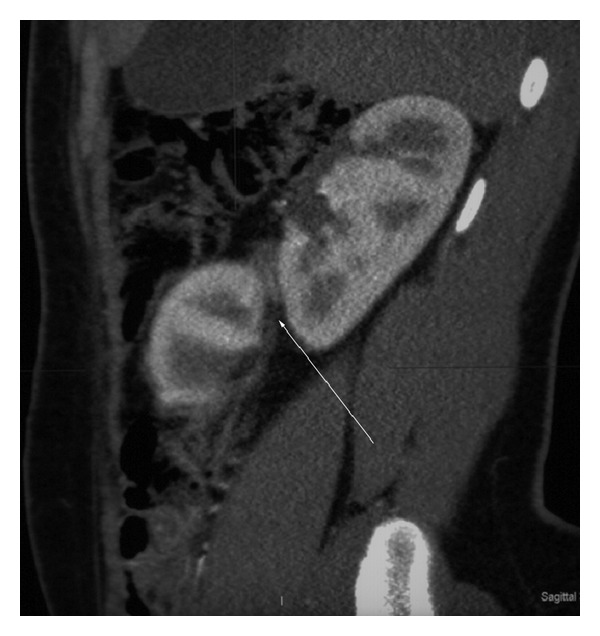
16-year-old adolescent male with left-to-right crossed renal ectopia. Sagittal CT scan with intravenous contrast, corticomedullary phase. Showing clear plane of separation between the two kidneys (white arrow), each kidney having its own Gerota's fascia.
